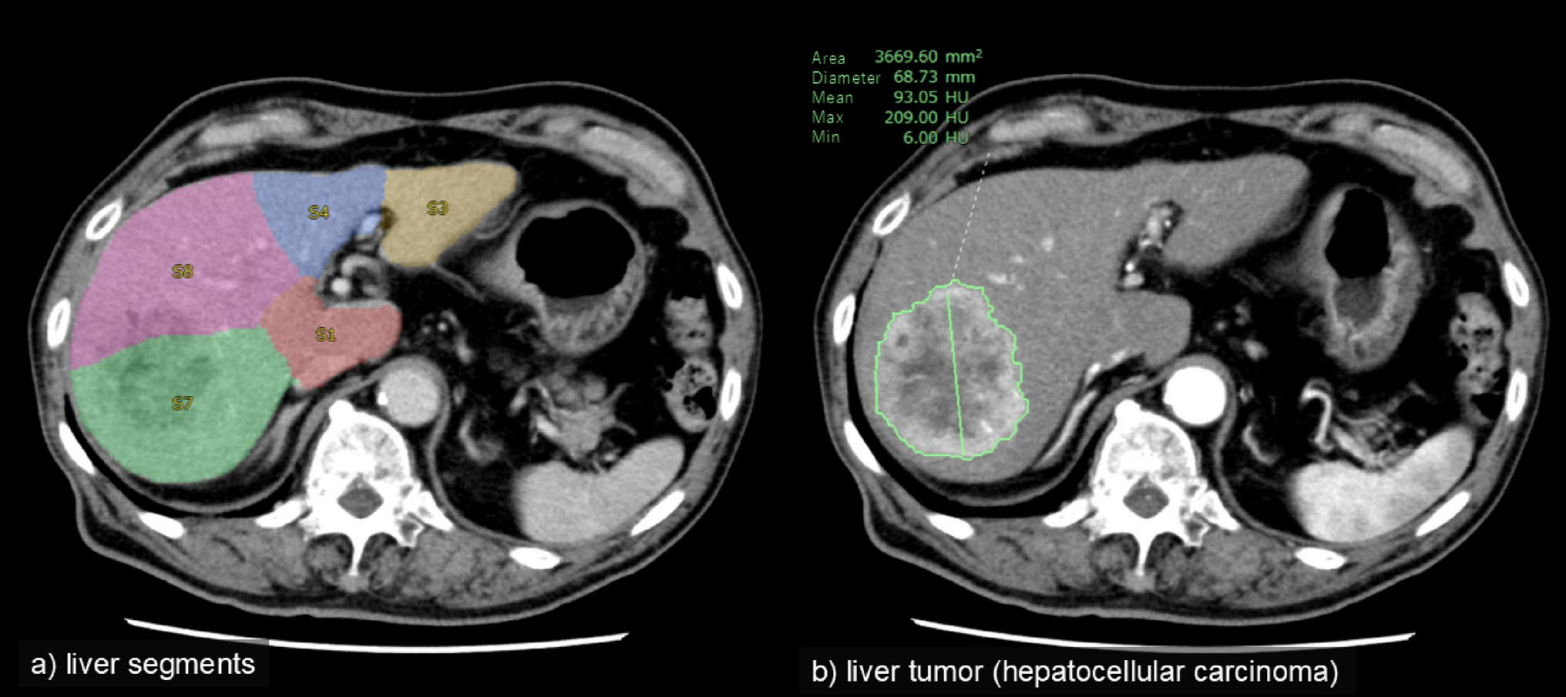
Segmentation using an AI-equipped radiological viewer. Radiological viewer with integrated AI features (SYNAPSE SAI viewer, Fujifilm; approved for clinical applications in Japan, not FDA-approved) provides reading support functions. a Automatic segmentation of liver segments, which is displayed as an overlay on CT images. b Manual input of two points on the margin of a liver tumor to extract the tumor region. This automated/semi-automated segmentation is useful for pre-treatment and post-treatment assessments. *AI* Artificial intelligence, *CT* Computed tomography
